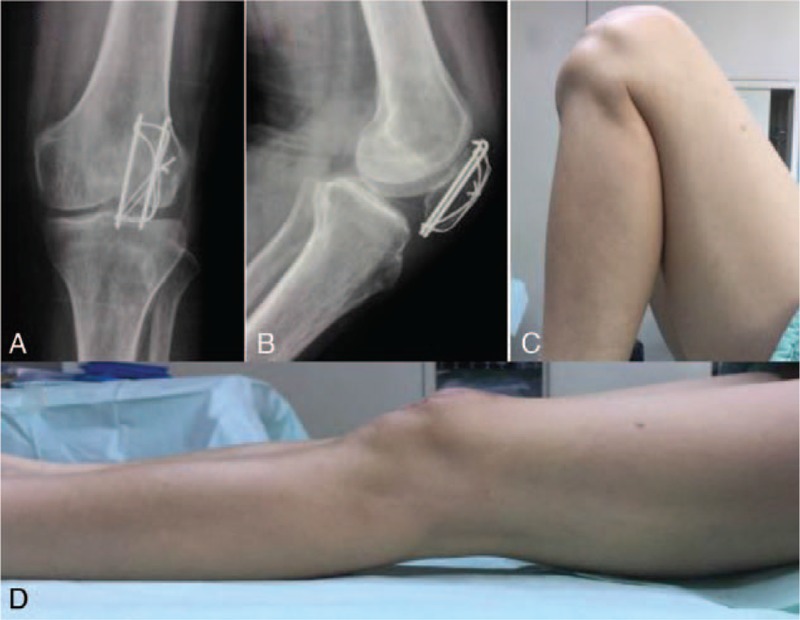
(A) Anteroposterior and (B) lateral radiographs at 1-year postoperatively showed perfect healing of the patella fracture. (C) and (D) Functional photograph of the left knee showing an excellent range of motion of the injury knee.
